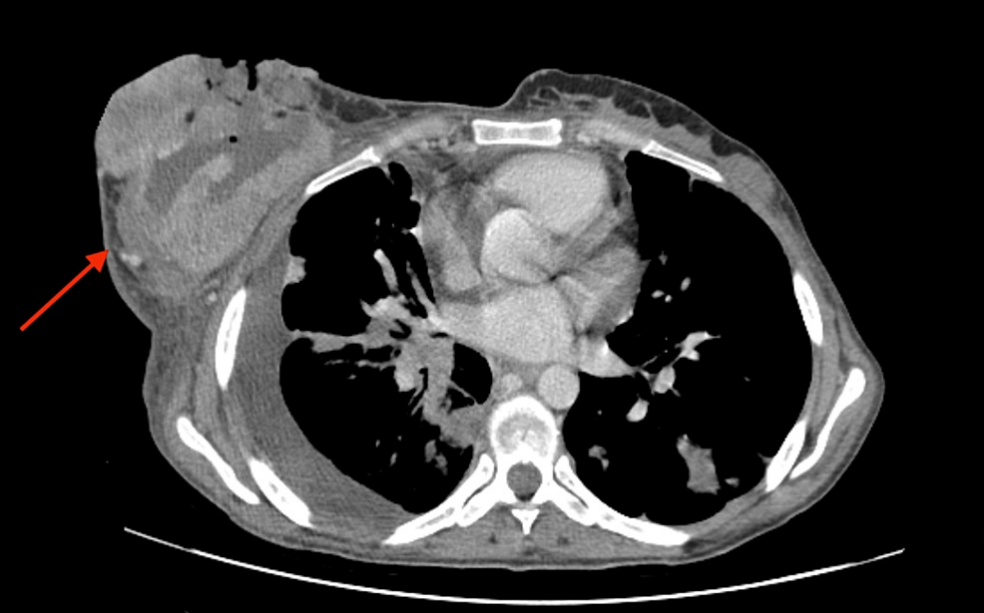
Contrast-enhanced thoracoabdominal CT A large neoformative lesion in the right breast (red arrow), ulcerated, with air-fluid levels inside.
